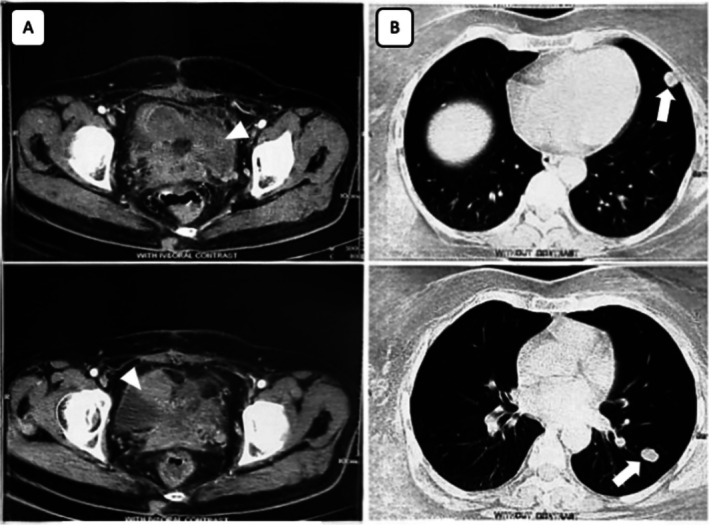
(A) Patient's postoperation CT of abdomen and pelvis revealed two large heterogeneous hyper‐enhancing adnexal lesions in the pelvic cavity in place of each ovary, measuring 5.5 × 8.3 cm on the right and 6.4 × 5.1 cm on the left (arrow heads). (B) CT of the chest revealed multiple nodular, hyper‐enhancing noncalcified lymphadenopathy measuring greater than 1.0 cm in size in various regions of the lungs and mediastinum, consistent with metastatic disease (arrows).
